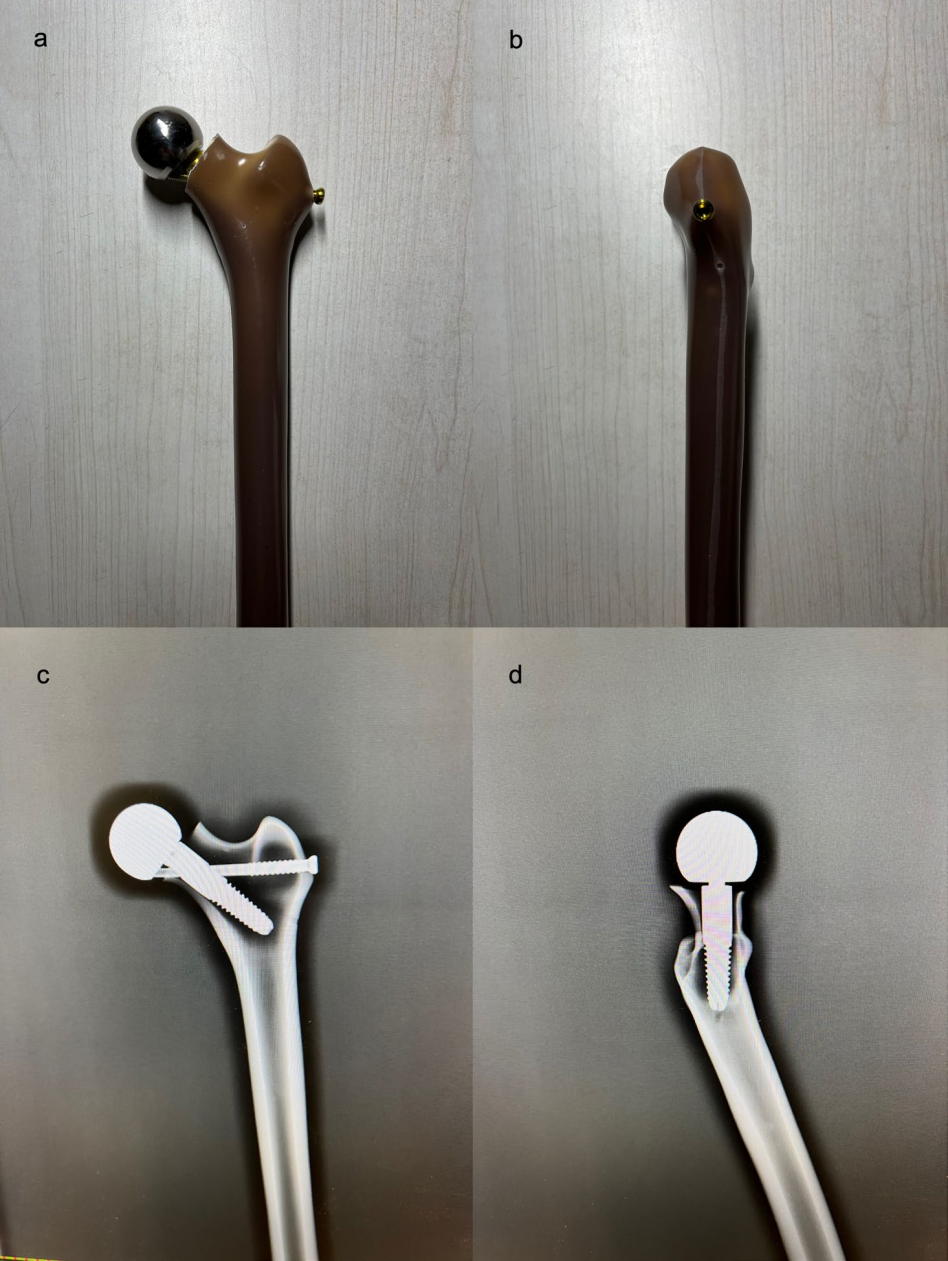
BHA implanted model preparation. (a) Frontal and (b) lateral profiles of BHA implanted model. (c) Frontal and (d) lateral radiographs of BHA implanted model.
